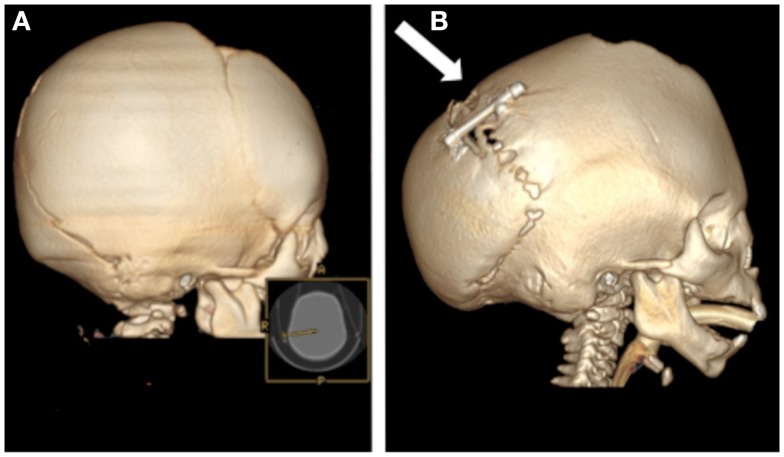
Computed tomography scans of an infant with multiple suture synostosis preoperatively (A) and 4 months after distraction of the posterior cranial vault (B). The distraction footplates have been gradually separated by a distance of 25 mm and evidence of calcified bony regenerate is present between the footplates (arrow).
